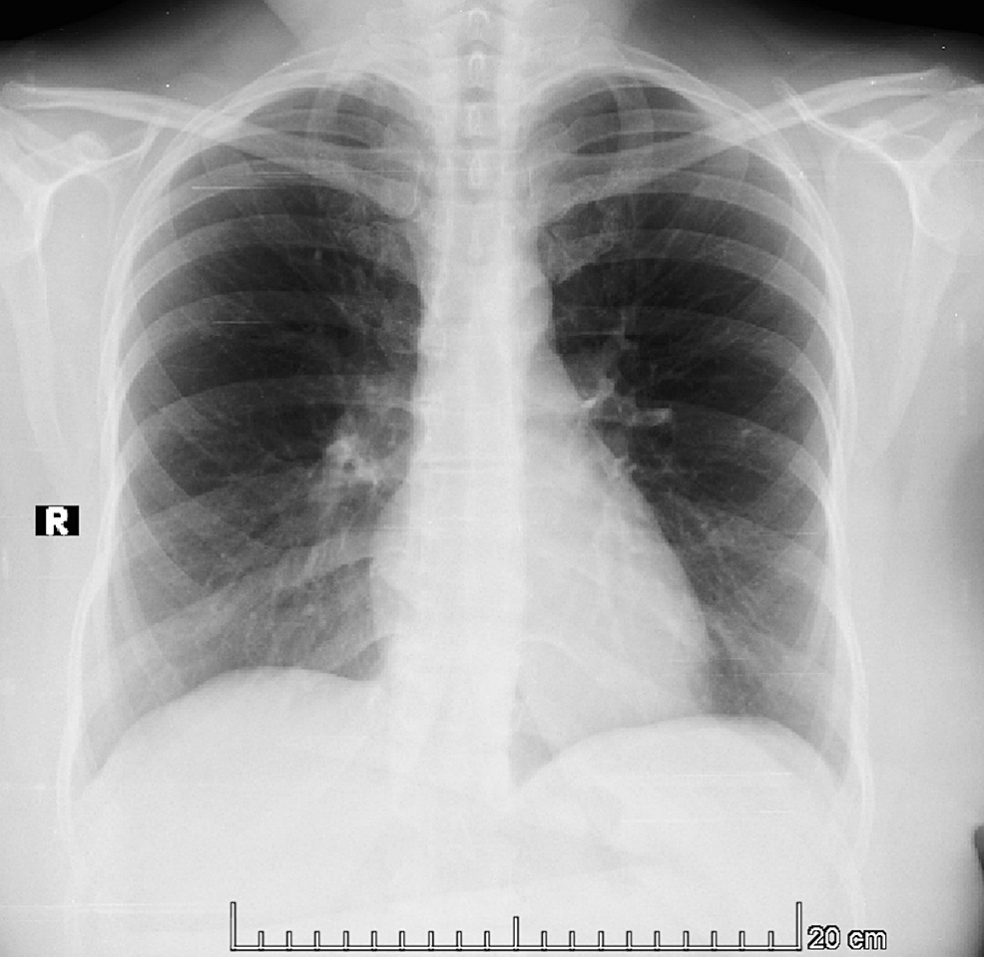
PA X-ray of the chest showed no consolidations, no infiltrations, and no granulomas at the apex.
